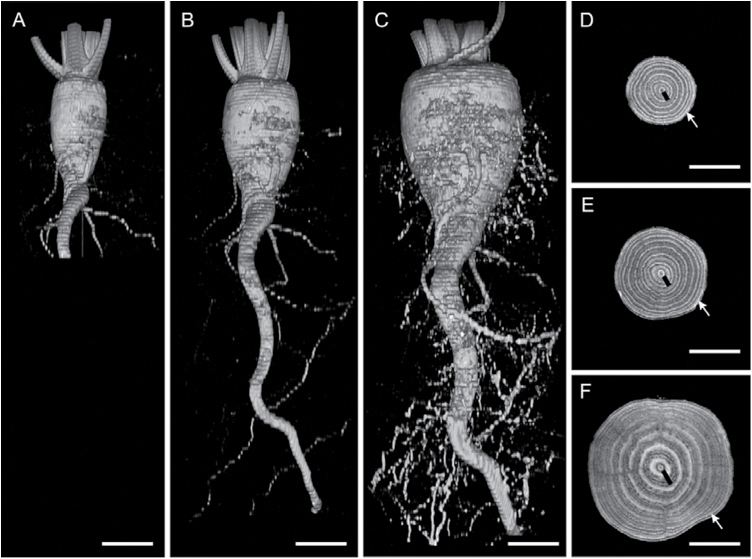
MRI images of a HS plant. The taproot of the sugar beet is shown in isosurfaces and cross-sections at 11 weeks (A, D), 17 weeks (B, E), and 25 weeks after sowing (C, F). (D, E, F) In the cross-sections, the thickness of the innermost cambial ring (no. 1) is marked with a black line; the outermost one with a white arrow. The scale of the isosurfaces and cross-sections is indicated by the white line (2cm). Taproots grew out of the field of view (70×70mm^2^) 16 weeks after sowing; therefore, two measurements per taproot, one set above the other, had to be conducted to capture the root system (B, C). The two MRI projections were concatenated together by image analysis.
